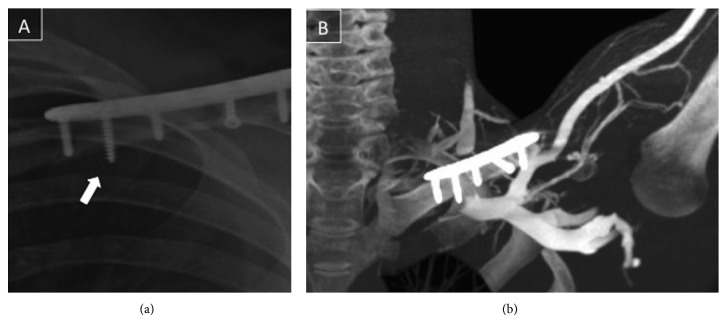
(a) Magnified image of the medial clavicle. Notice that the second screw from medial to lateral has 3 spirals protruding from the inferior face of the clavicle. See white arrow. (b) A dynamic angio-computed tomography scan revealed external compression of the subclavian vein in contact with the second screw from medial to lateral while the patient had his arm raised. See white arrow.
